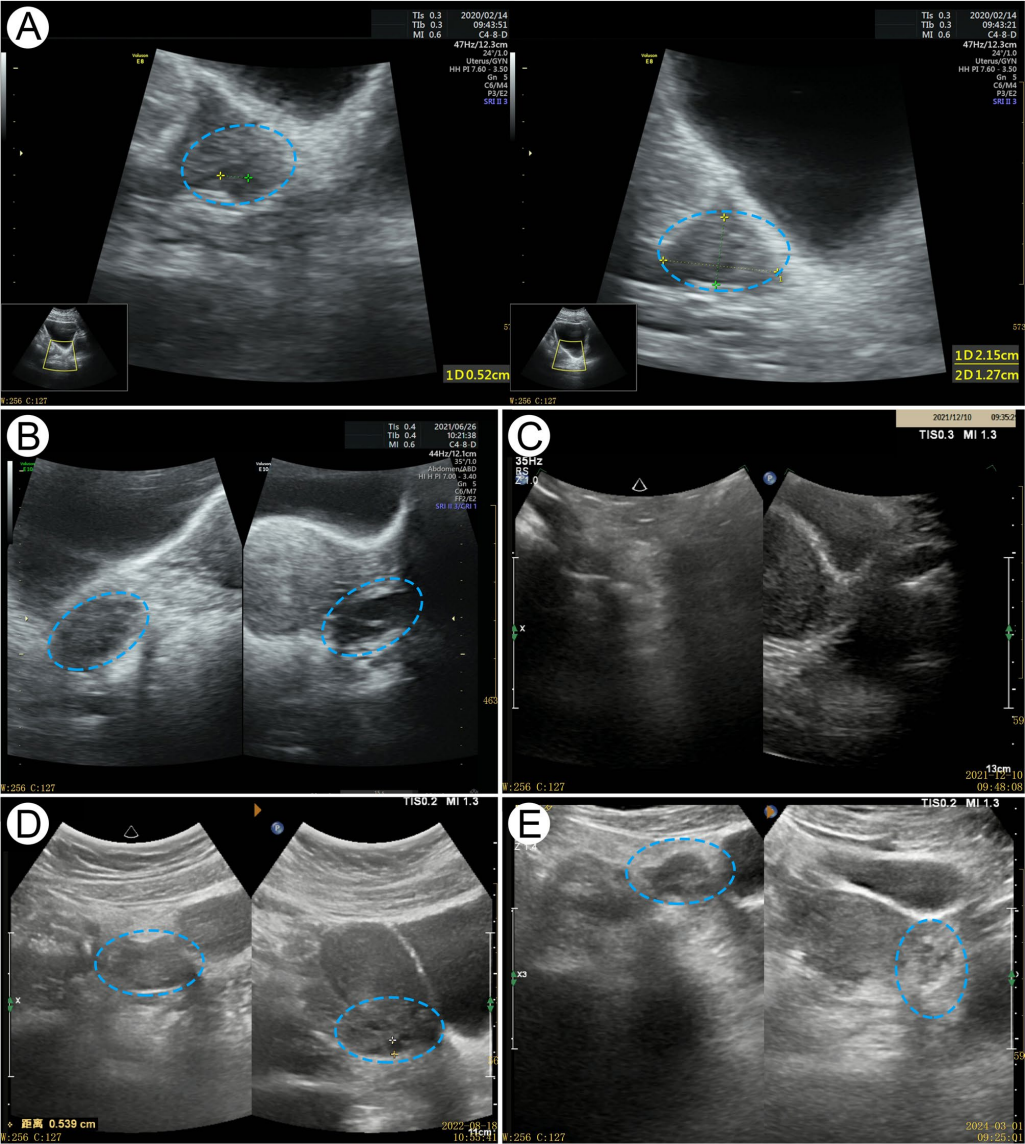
Ultrasound pictures of the patient’s ovarian. The blue frame indicates the changes in the patient’s ovaries: A Baseline (2020.02.14): Bilateral ovaries with few follicles (2–3 per side). B Before discontinuation of Heyan Kuntai capsule (HYKT) (2021.6.26): The right ovary measured 2.7 × 1.8 × 2.5 cm with 5–6 follicles (max 0.7 cm), and the left ovary measured 2.6 × 1.2 × 2.0 cm with 4–5 follicles (max 0.9 cm). C After discontinuation of HYKT (2021.12.10): Ovaries shrank with no visible follicles. D After adding HYKT (2022.8.18): Unilateral antral follicles and ovarian volume increased. E Final follow-up (2024.3.1): The right ovary (5–6 follicles, max 0.7 cm) and the left ovary (4–5 follicles, max 0.5 cm) were normal in size
